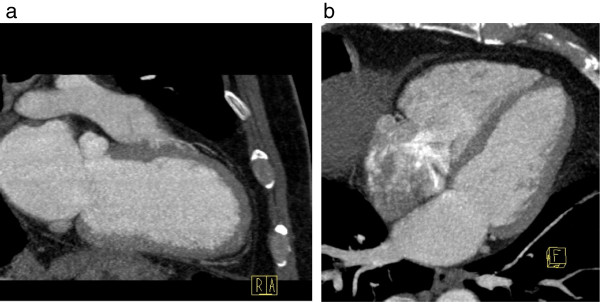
Computed tomography. (a) Two-chamber and (b) four-chamber axial view images performed 12 months prior to clinical attendance. No mass is seen. R indicates Right, A anterior and F Foot.
